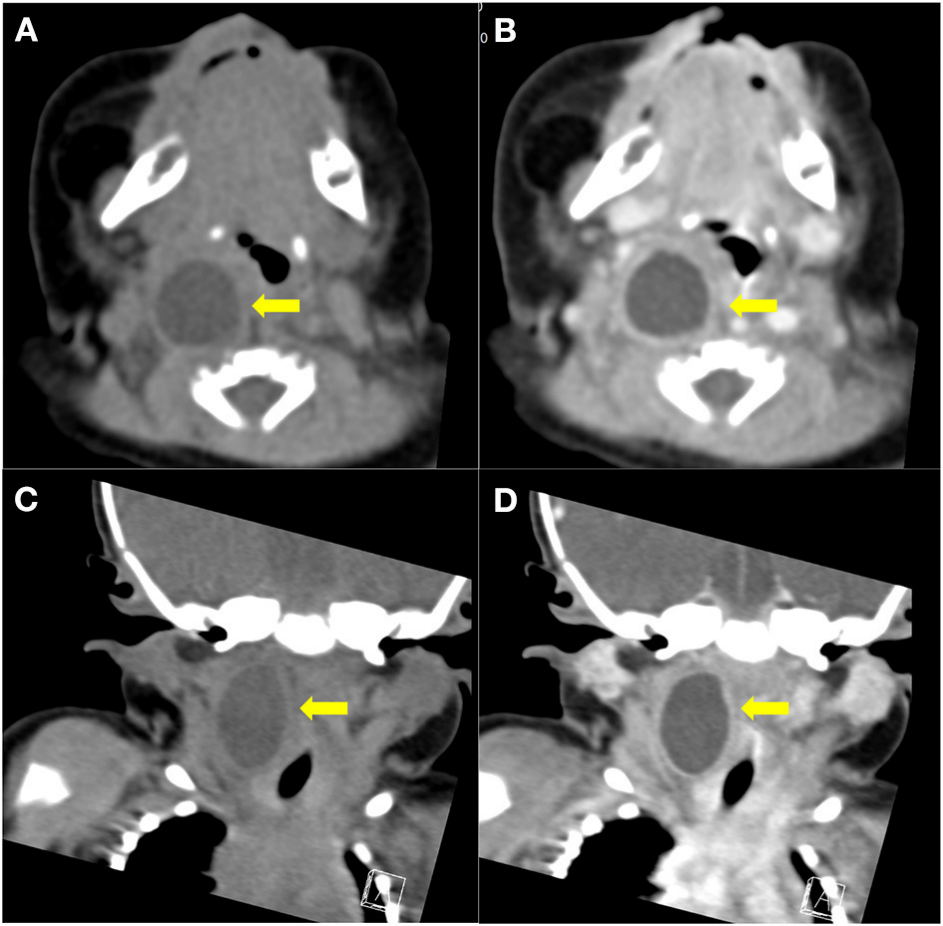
Cervical computed tomography in the patient at the age of 2 days. Coronal and sagittal imaging of cervical computed tomography (CT) revealed that the cyst (2.7 × 1.6 × 1.7 cm) was located in the parapharyngeal space close to the esophagus, and was causing mild compression of the trachea and cervical vessels (A,C). No reinforcement was observed after contrast-enhanced CT scanning (B,D).
